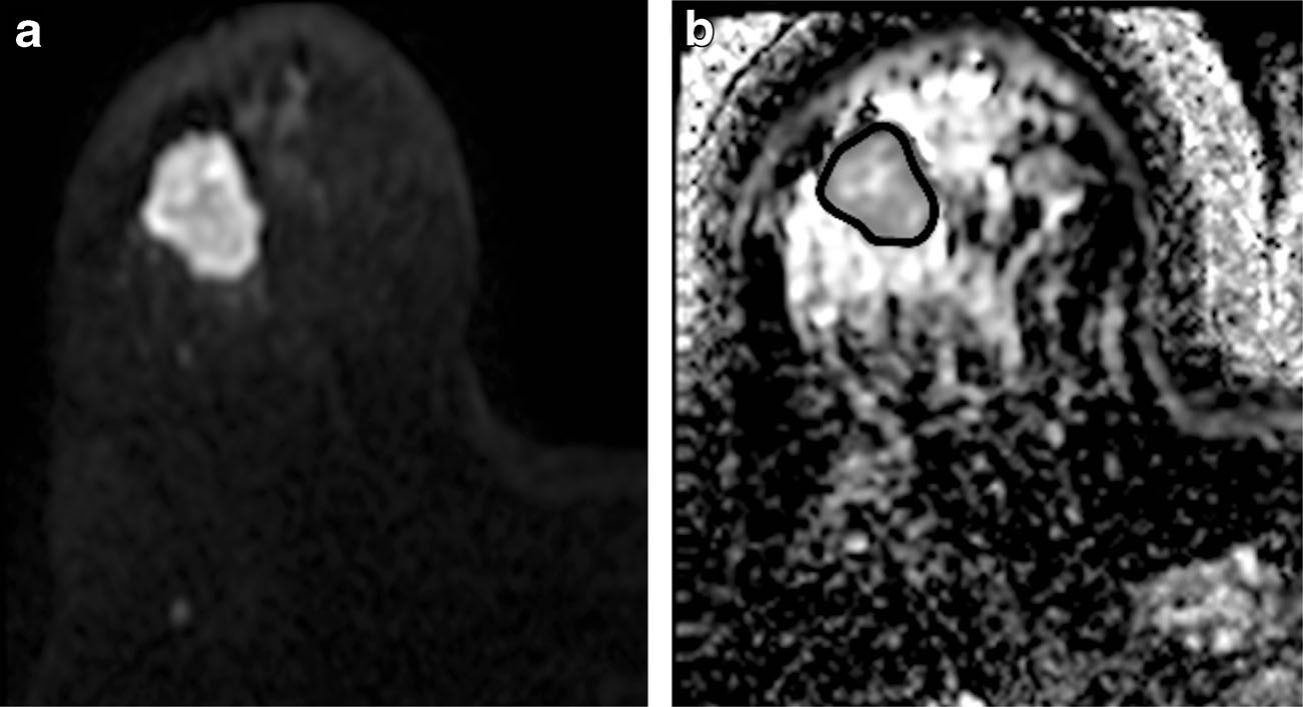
Representative images from pretreatment MRI of a 49-year-old female patient with cancer of the right breast: a axial DW image showing hyperintense tumour with restricted diffusion on the *b*900 s/mm^2^ image. b ADC map was generated from two *b* values (0, 900 s/mm^2^). ROI was drawn on the primary lesion and copied to the ADC map (ADC = 0.92 ± 0.094 × 10^−3^ mm^2^/s)
